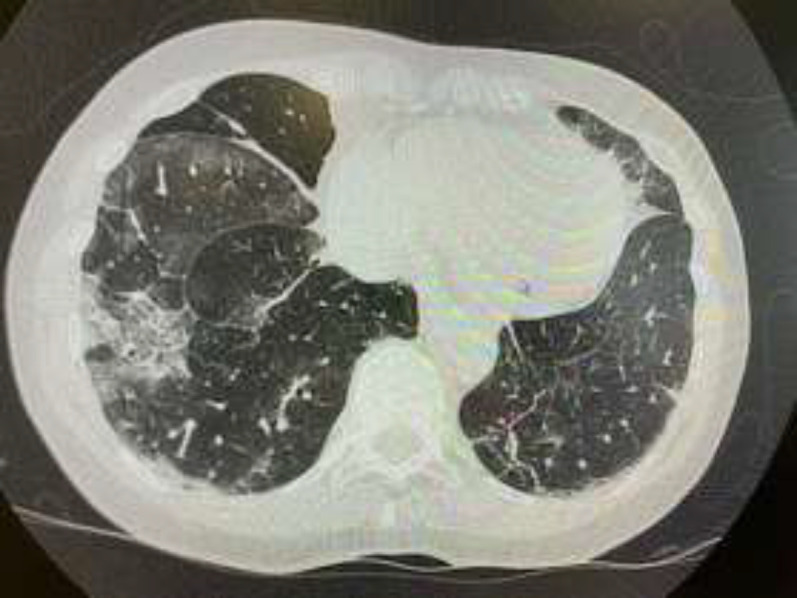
Chest CT scan showing right lower pulmonary opacity
